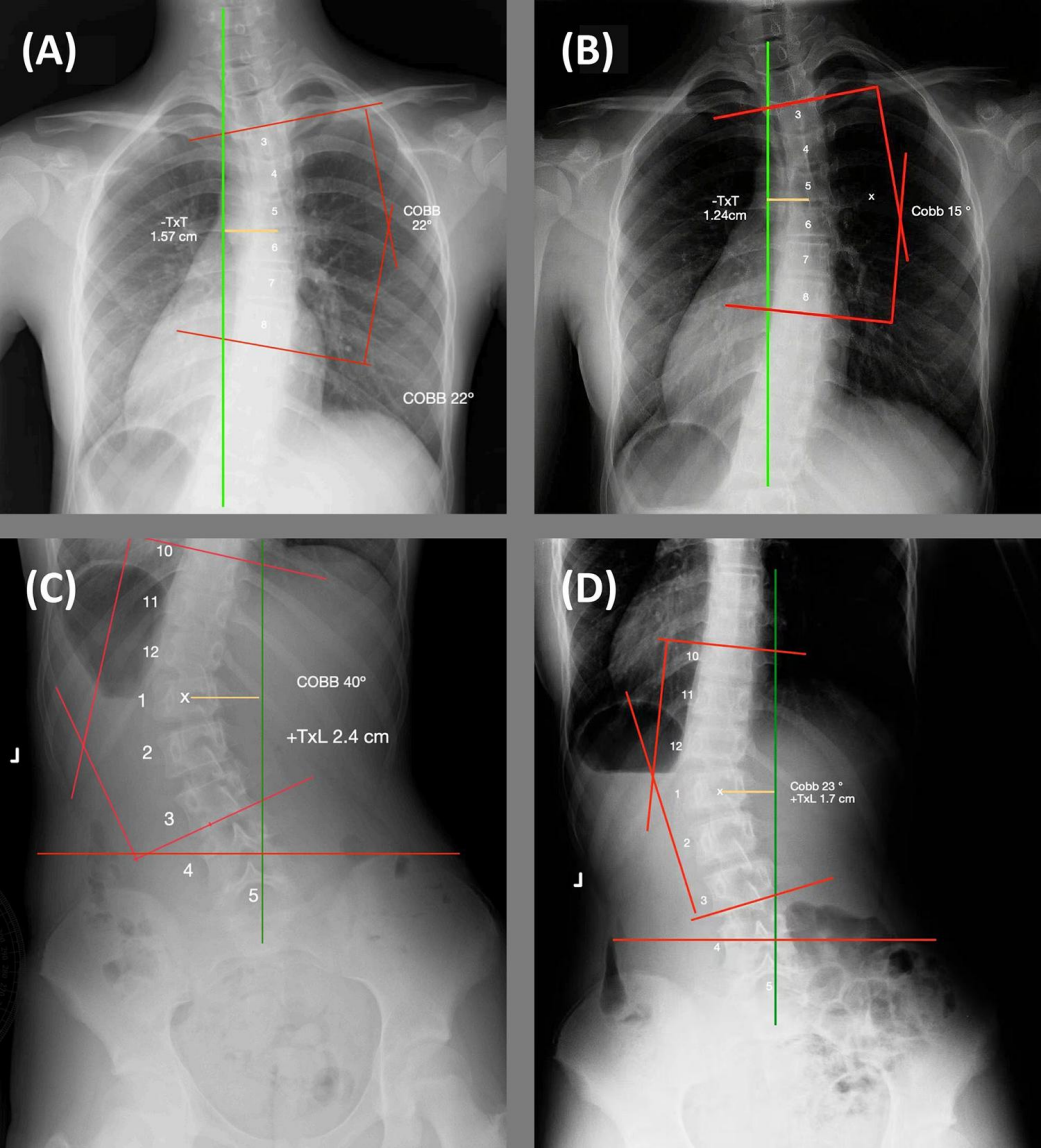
Comparison of initial x-rays and x-rays at 32-week assessment: (A) initial thoracic cobb angle 22° in x-ray; (B) initial lumbosacral cobb angle 40° in x-ray; (C) 32-week thoracic cobb angle 15° in x-ray; (D) 32-week lumbosacral cobb angle 23° in x-ray.
